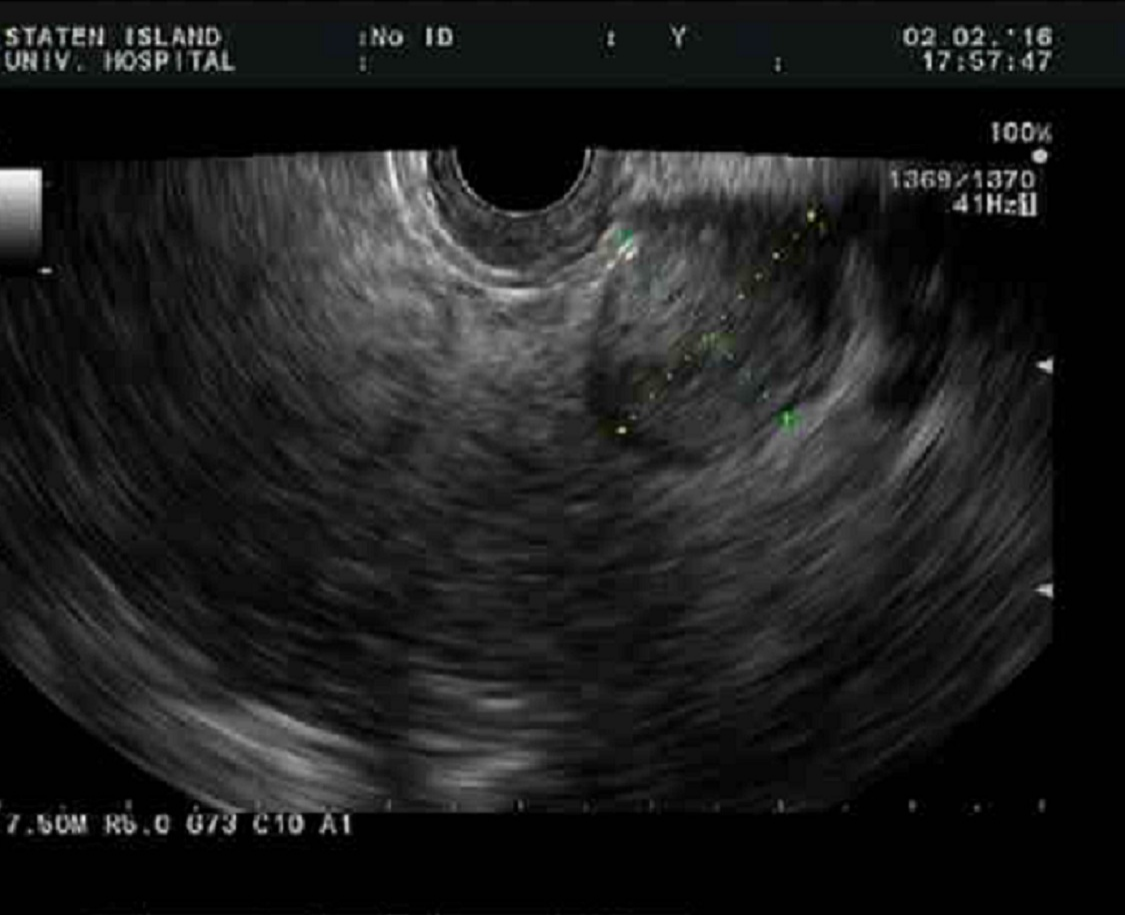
Endoscopic ultrasound Endoscopic ultrasound image showing 2.1 x 1.8 cm hypoechoic pancreatic tail mass
